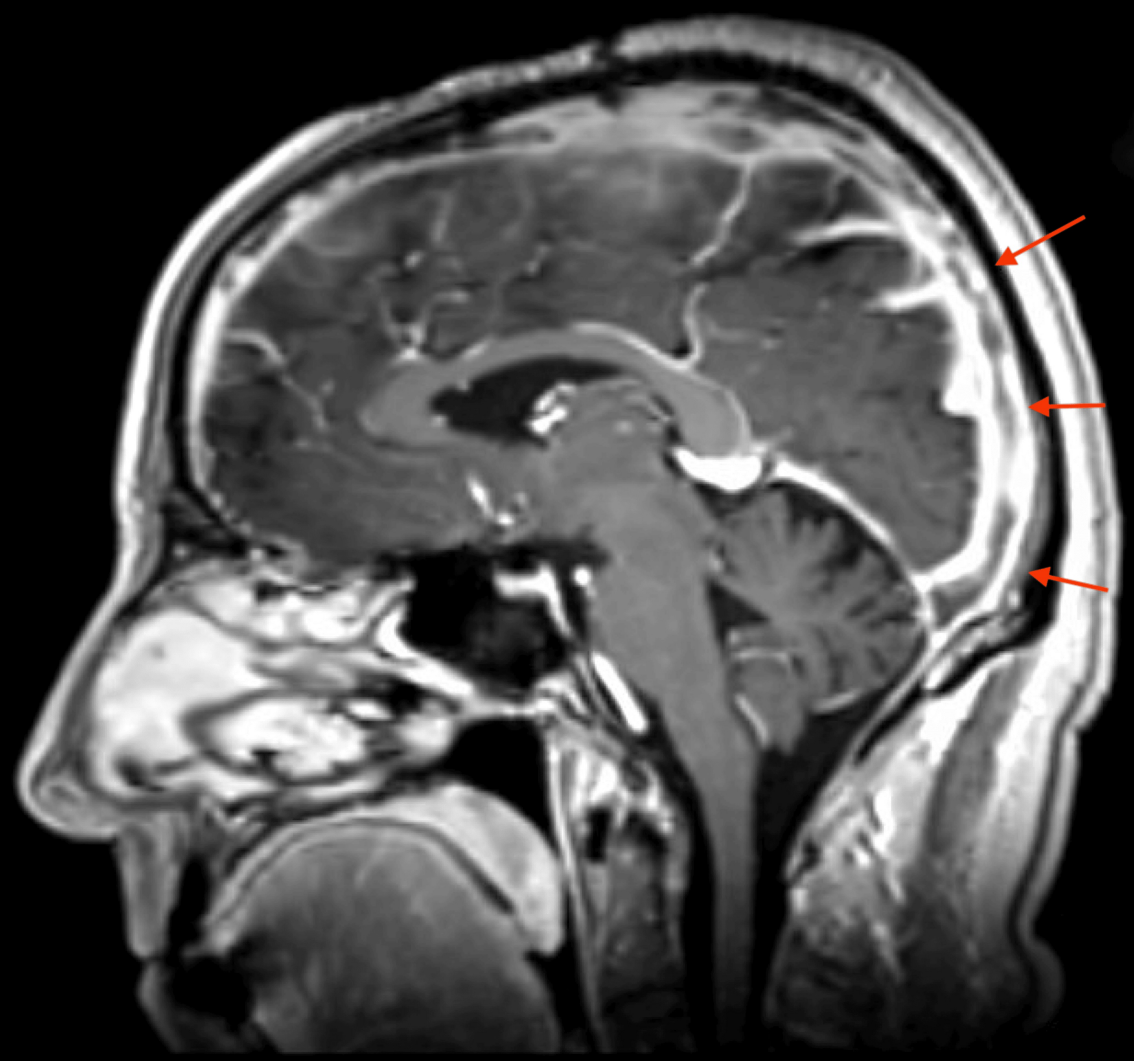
MRI of the brain with contrast, sagittal view, demonstrating multisinus involvement including the superior sagittal sinus (arrows)
